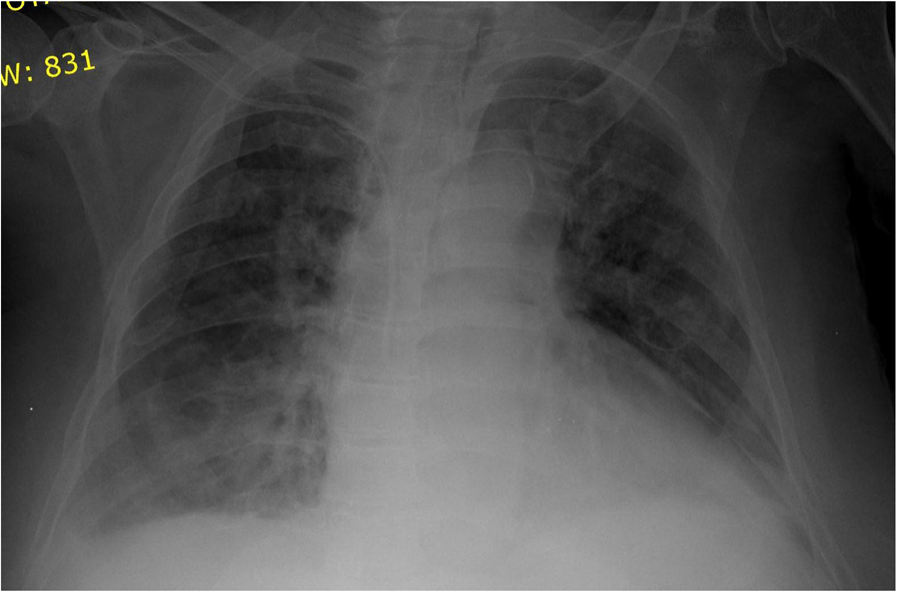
Chest radiograph shows two fractures of fifth and sixth ribs on the right and a cavitary lesion with an air-fluid level in the basal segment of the right lower lobe.
